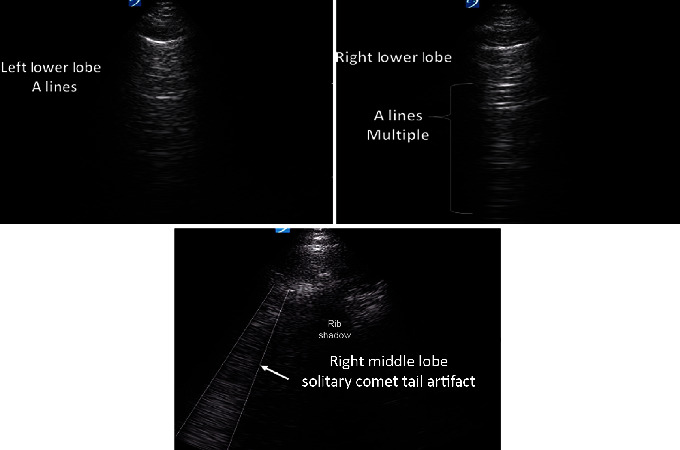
The patient's bedside ultrasound images after extubation revealed horizontal artifact consistent with normal lung parenchyma, and only a single comet tail artifact noted after bedside ultrasound of all lung fields.
